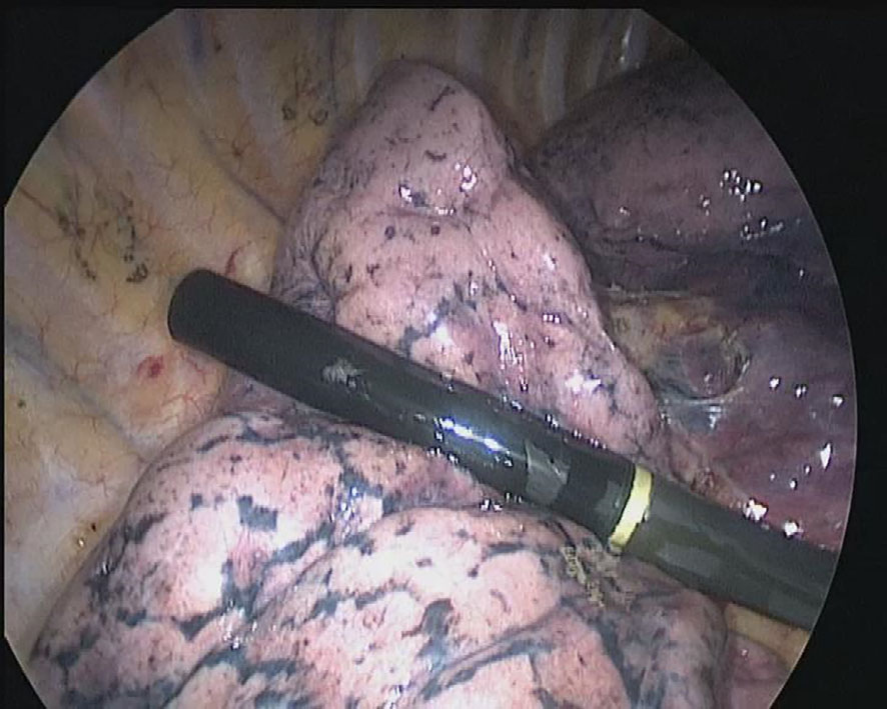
Intraoperative ultrasound localization of pulmonary nodules.
